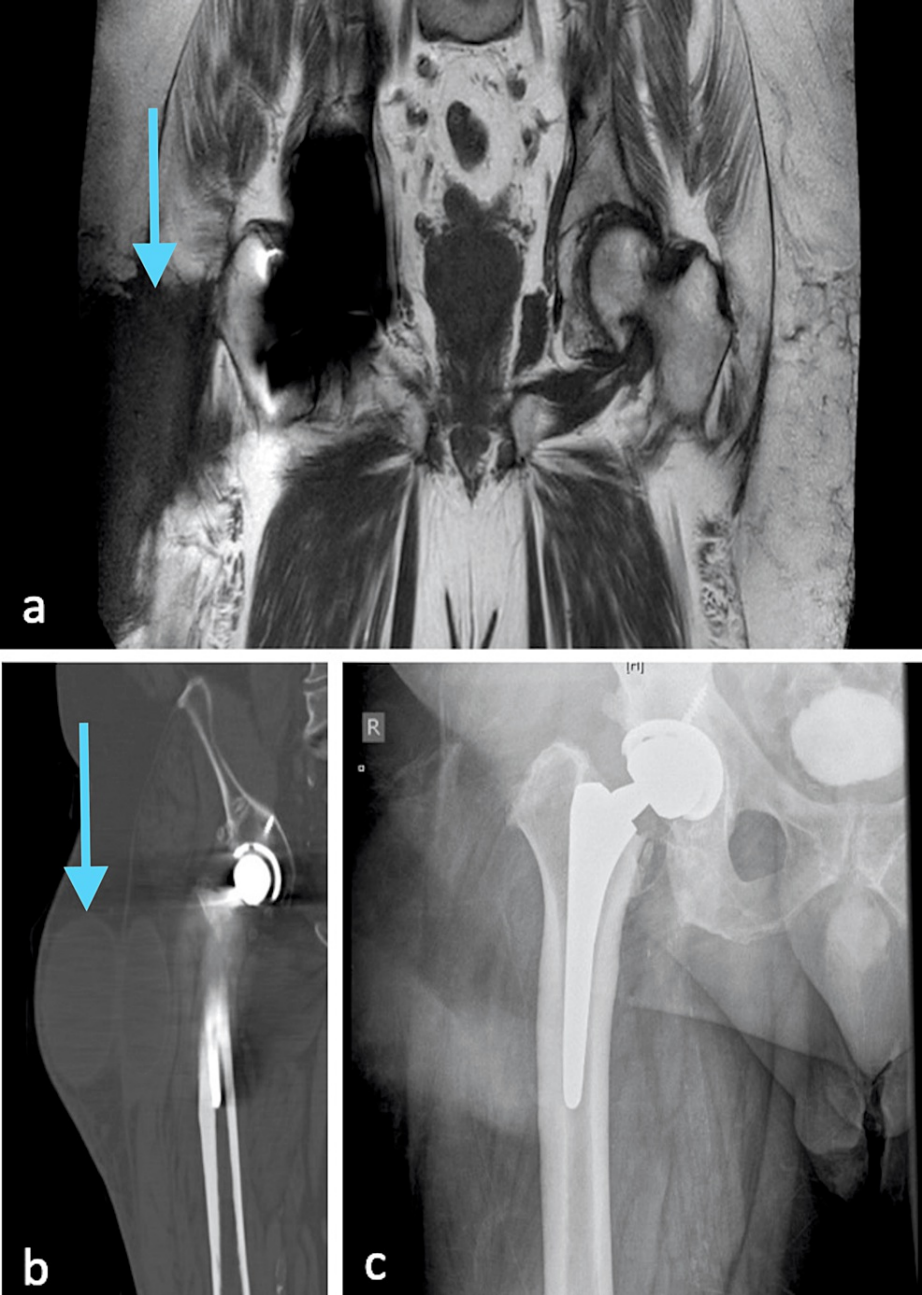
Preoperative imaging MARS-MRI shows a large lobulated cystic mass in the subfascial and extrafascial planes (a). Coronal CT shows the same large lobulated cystic mass (b) and anterior-posterior x-ray (c).
